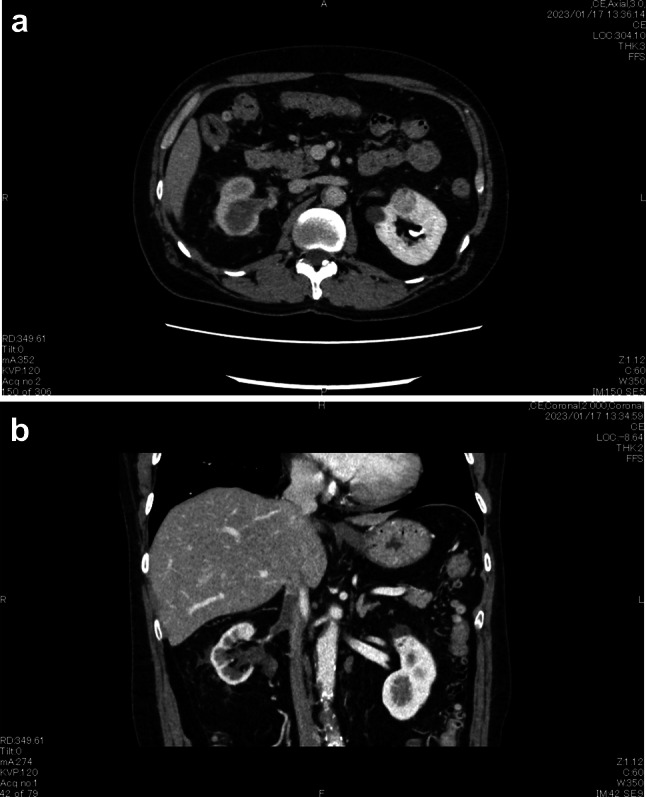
CT images of the right renal tumor after nivolumab plus ipilimumab (1 year after presentation). a The primary tumor has shrunk to 41 × 40 mm. b The maximum diameter of the thrombus is 8 mm and its length is 26 mm after the chemotherapy
